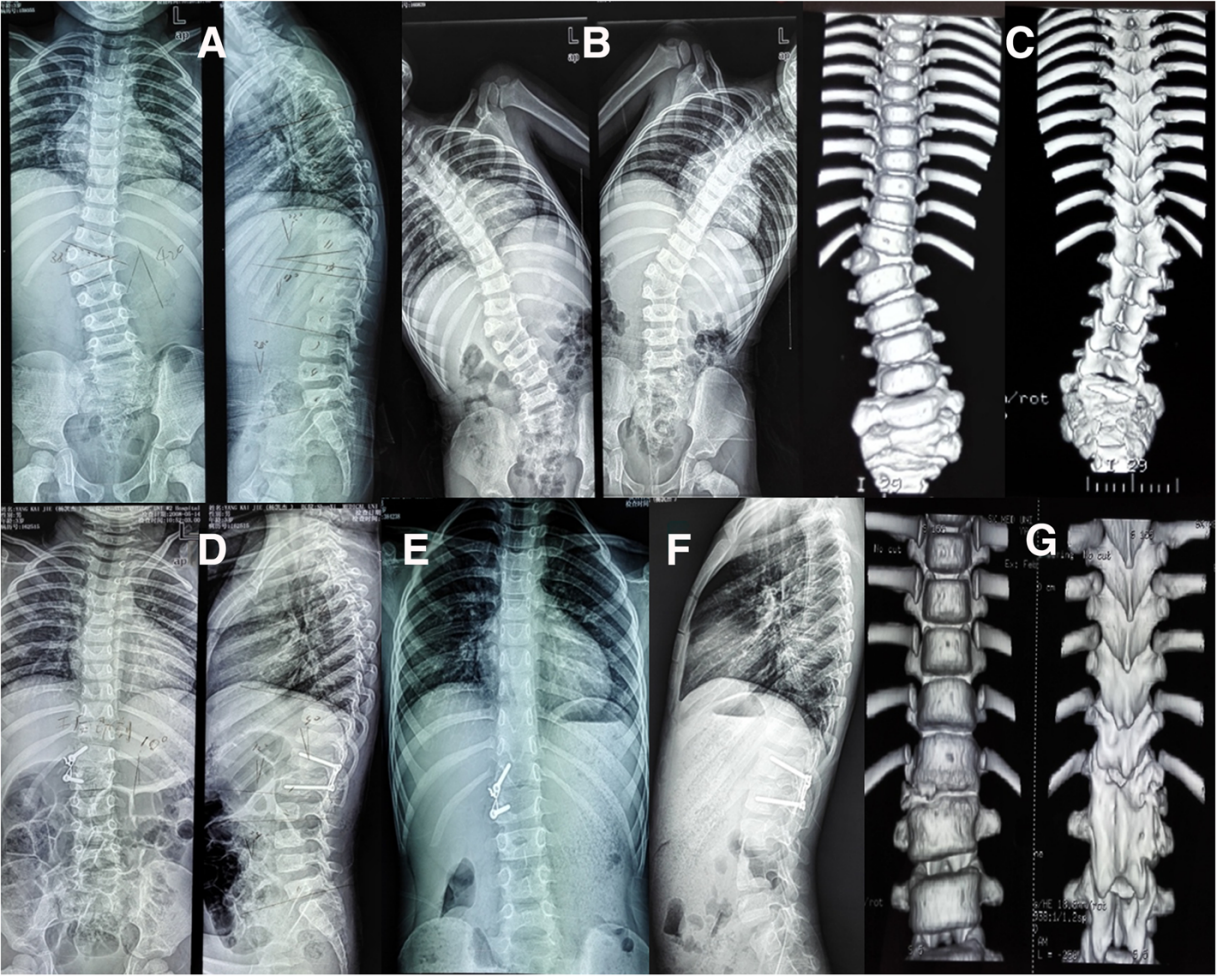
A 2 years 9 months old girl with T12 hemivertebra. Radiograph images and 3D-computed tomography images obtained preoperatively (a–c), postoperatively (d), and at the latest follow-up visit, 69 months later (e, f, g)
